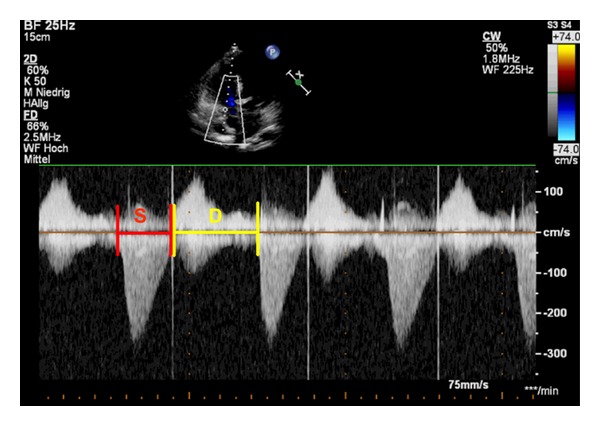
Apical 4-chamber view. Doppler-derived tricuspidal regurgitation time (TR) interval ratio of systolic (S) duration to diastolic (D) duration (S/D). The ratio of S/D duration was calculated by dividing the duration of the TR spectral Doppler flow pattern by the time interval of the cardiac cycle that did not include TR. Systolic and diastolic duration using TR duration was measured by CW-Doppler from the apical 4-chamber view to calculate the S/D ratio. The red lines show the systolic duration, and the yellow lines show the diastolic duration.
